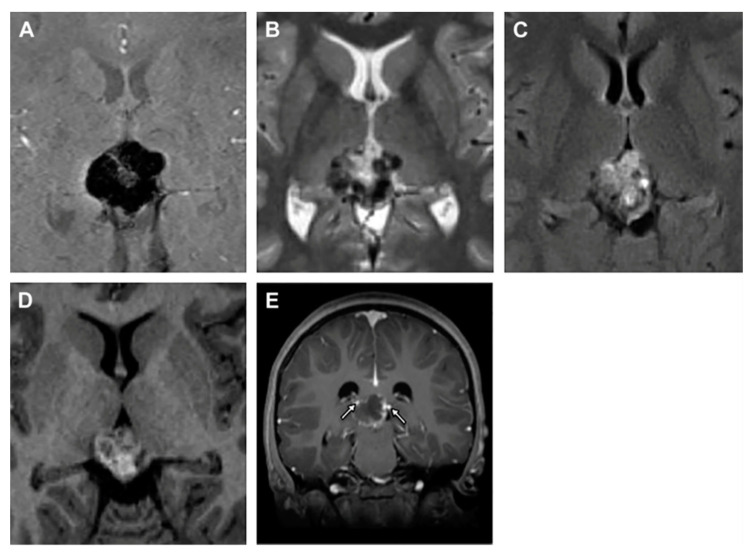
Pineal choriocarcinoma MRI. Susceptibility-weighted imaging (A), T2WI (B) and pineal heterogeneous mass on T1WI (C,D). The arrows identify the faint peripheral enhancement after the intravenous administration of gadolinium (E). Reprinted with permission from Causil et al. (2016) [46]. Elsevier—2021 (License Number: 5034730033295).
